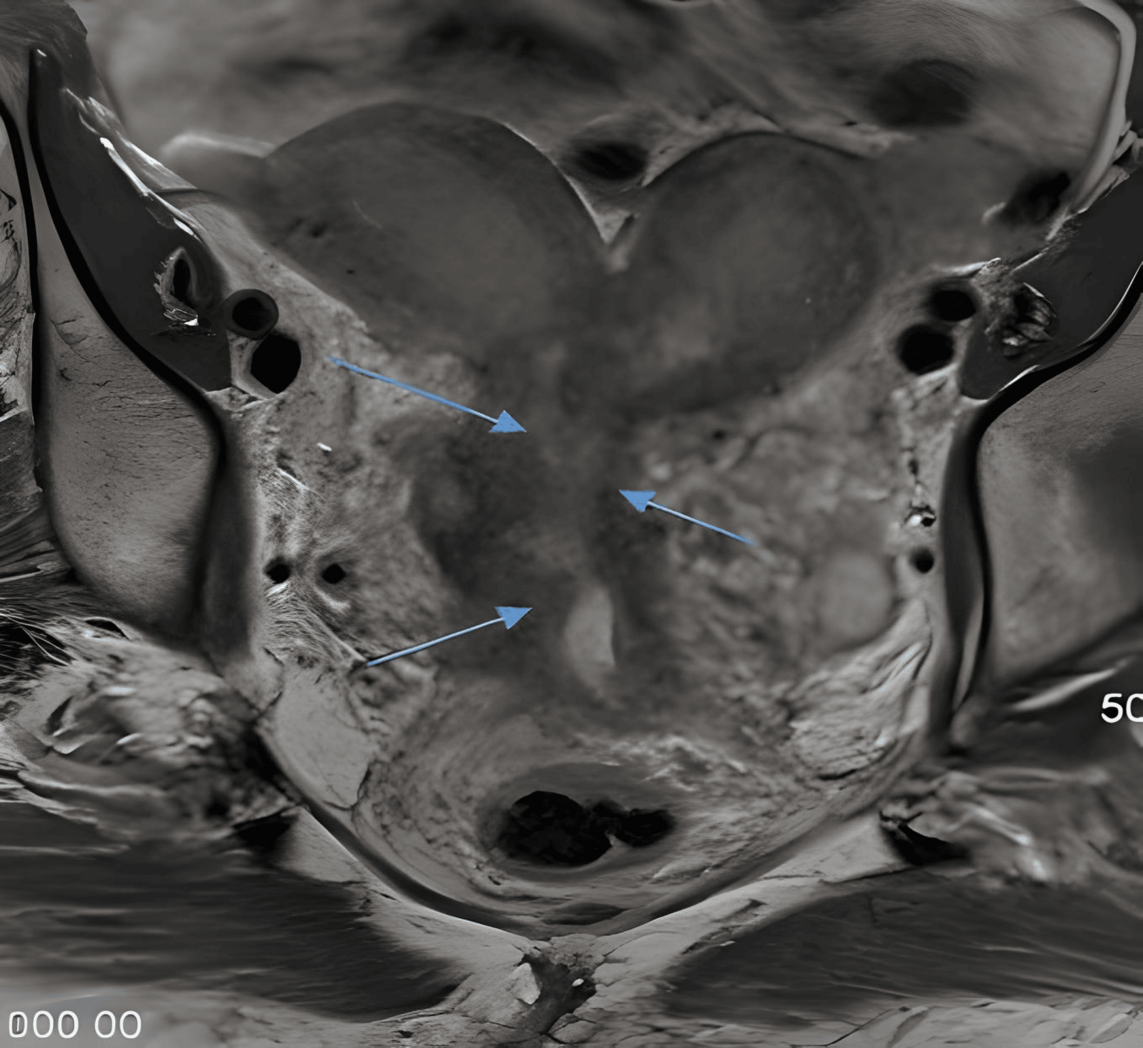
MR T2 coronal view Coronal MRI at diagnosis showing cervical tumor (blue arrows) invading bladder neck, upper vagina, and upper urethra and bicornuate uterus.
